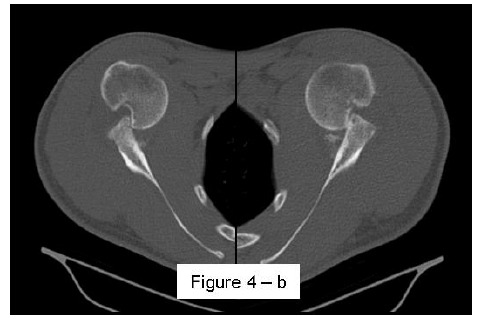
CT axial view with visualization of bilateral engaging wide and deep Hill-Sachs lesion 89x60mm (150 x 150 DPI).
